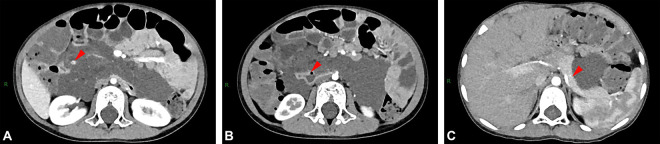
(Case 4): CT reveals a cystic lesion in the right mid-upper abdomen with heterogeneous density, containing multiple linear septations and calcifications (a, red arrowhead); the lesion envelops the duodenum (b, red arrowhead) and extends anteriorly to the front of the pancreatic body and tail (c, red arrowhead), suggestive of a lymphangioma.
